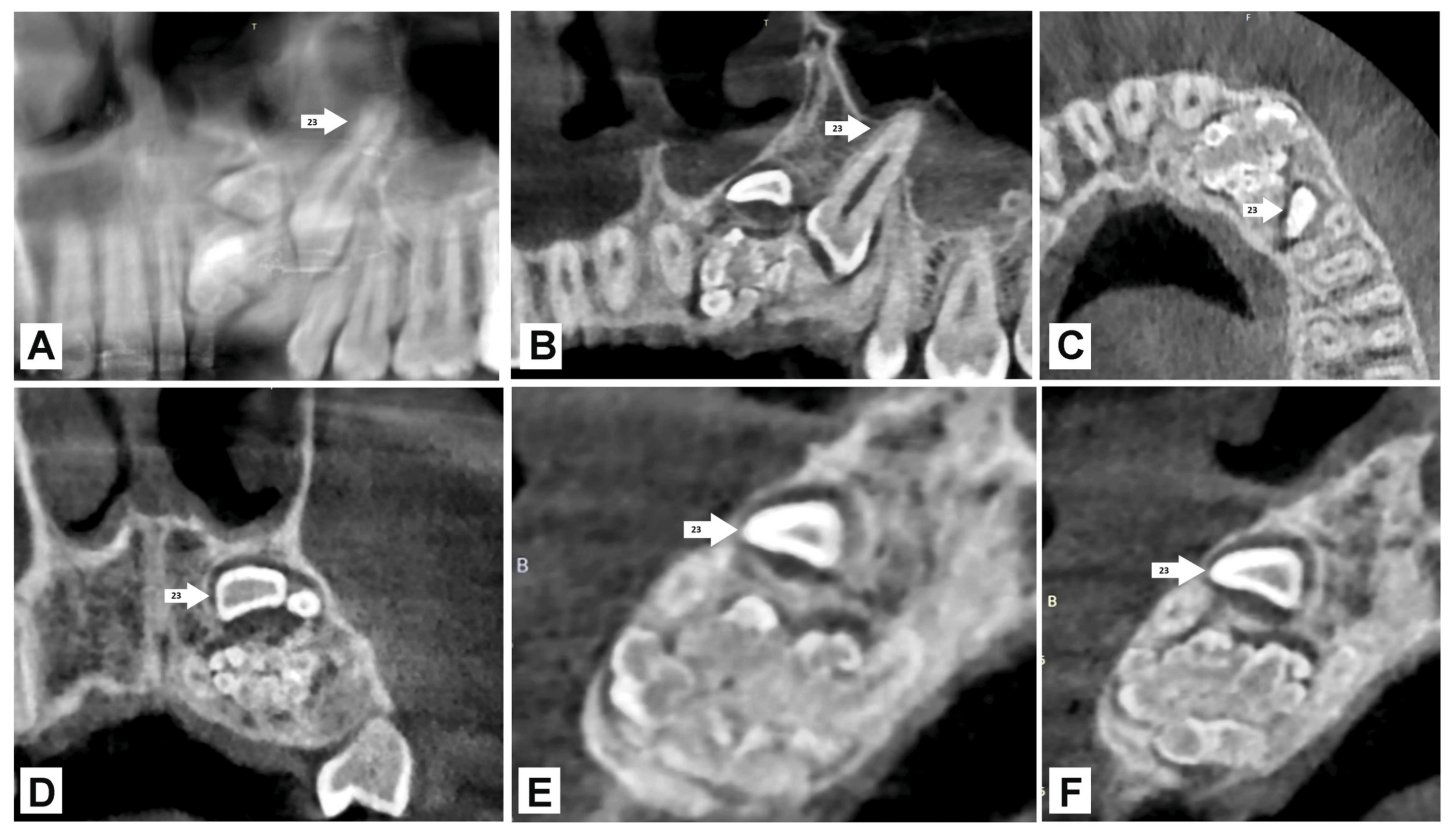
Preoperative CBCT with 22, 23 regions: A) Panoramic image, B) coronal section, C) axial, D) coronal section, E) cross section, and F) cross section Image credit: Netal Kela CBCT: Cone-beam computed tomography
